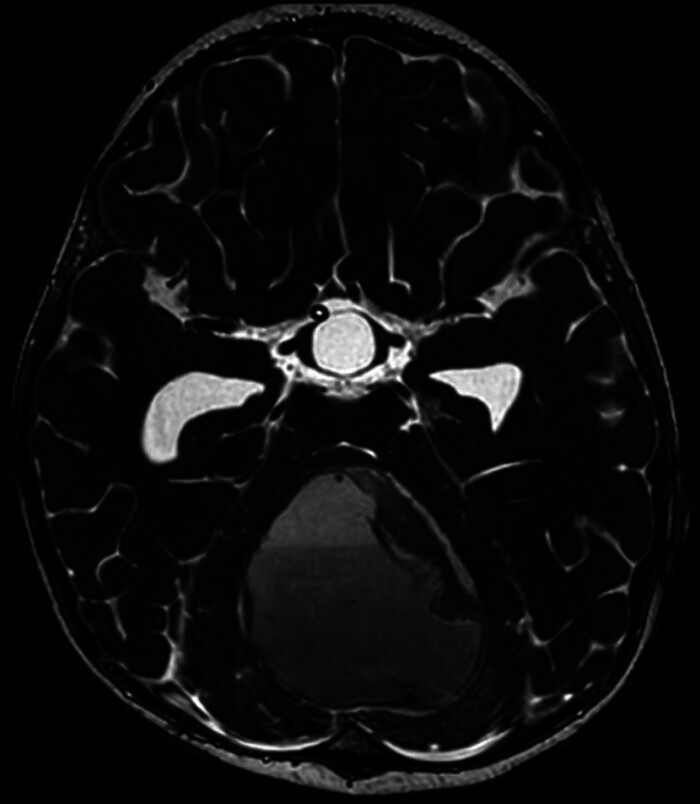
MRI demonstrating a large cystic mass in the posterior cranial fossa.
